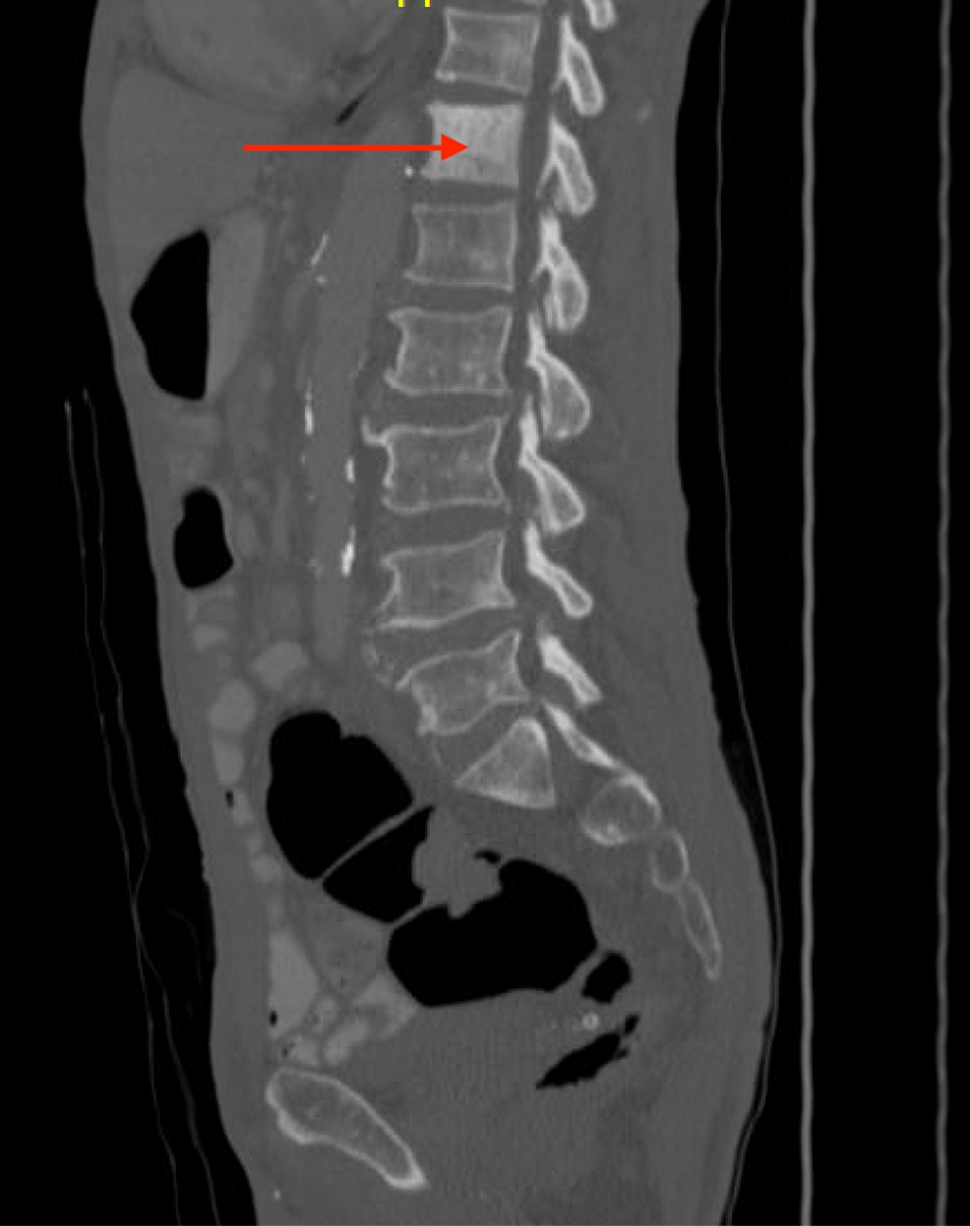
The arrow shows T12 sclerosis on CT scan.
